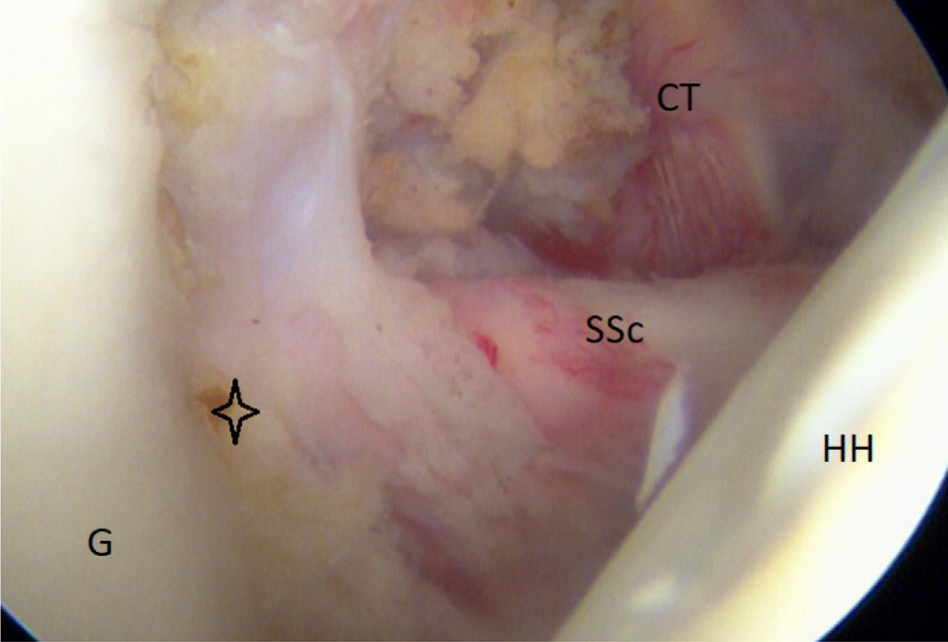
Arthroscopic view of a right shoulder, posterior viewing portal. The star sign indicates the lost anterior labrum on the glenoid (G). (CT conjoined tendon; HH, humeral head; SSc, subscapular tendon.)
